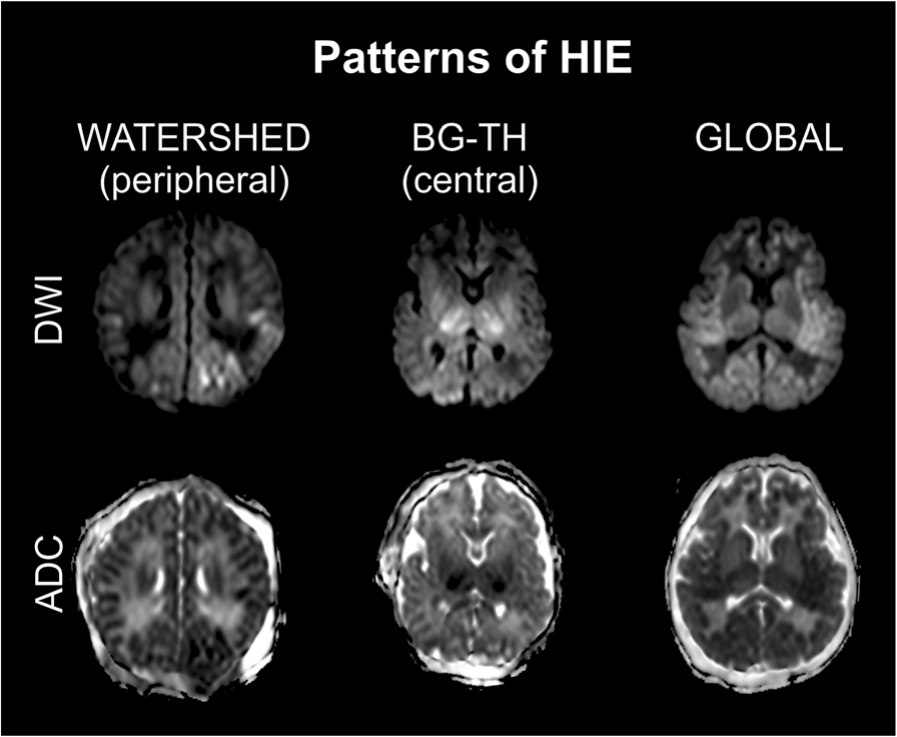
Patterns of HIE. Watershed (peripheral) type of injury, diffusion restriction bilaterally; Basal ganglia – thalamus (central) pattern, restricted diffusion in bilateral thalami and putamina; Global diffusion restriction on DWI and ADC
